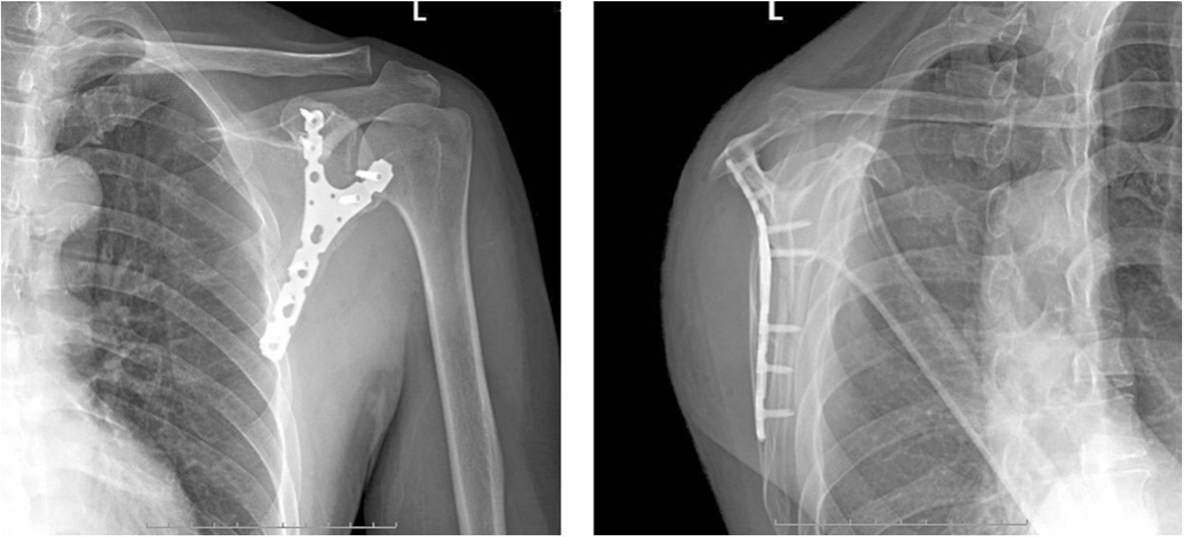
Postoperative scapular X-ray radiographies of the implanted Y-type locking plate in the anteroposterior and lateral positions
